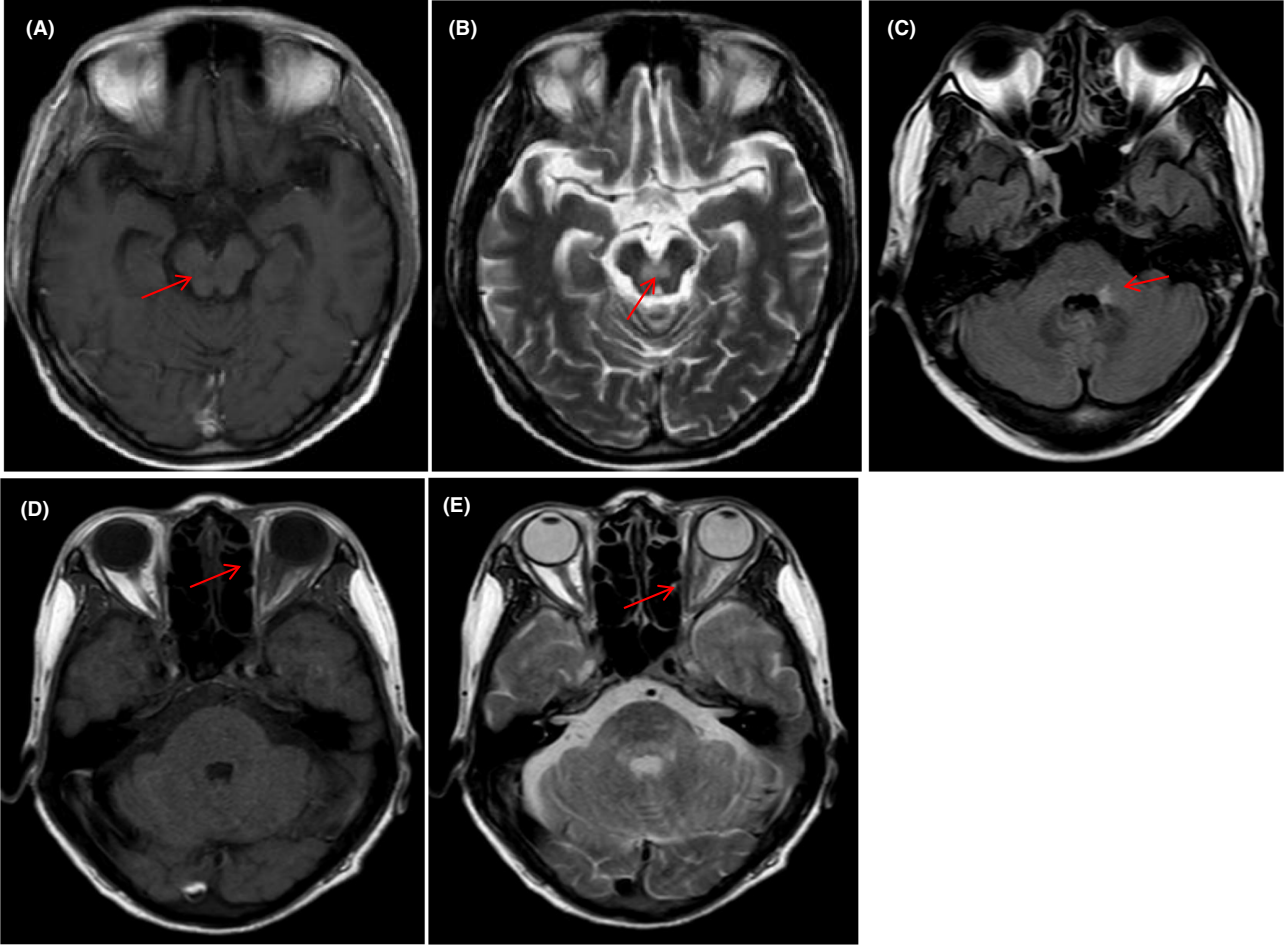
Initial brain MRI shown intracranial extra‐axial lesions on brain stem (A, B) and left pons (C), both hyposignal on T1 WI, T2 WI. (C and D) show ill‐defined lesion in left orbit on T1 WI, T2 WI, respectively.
